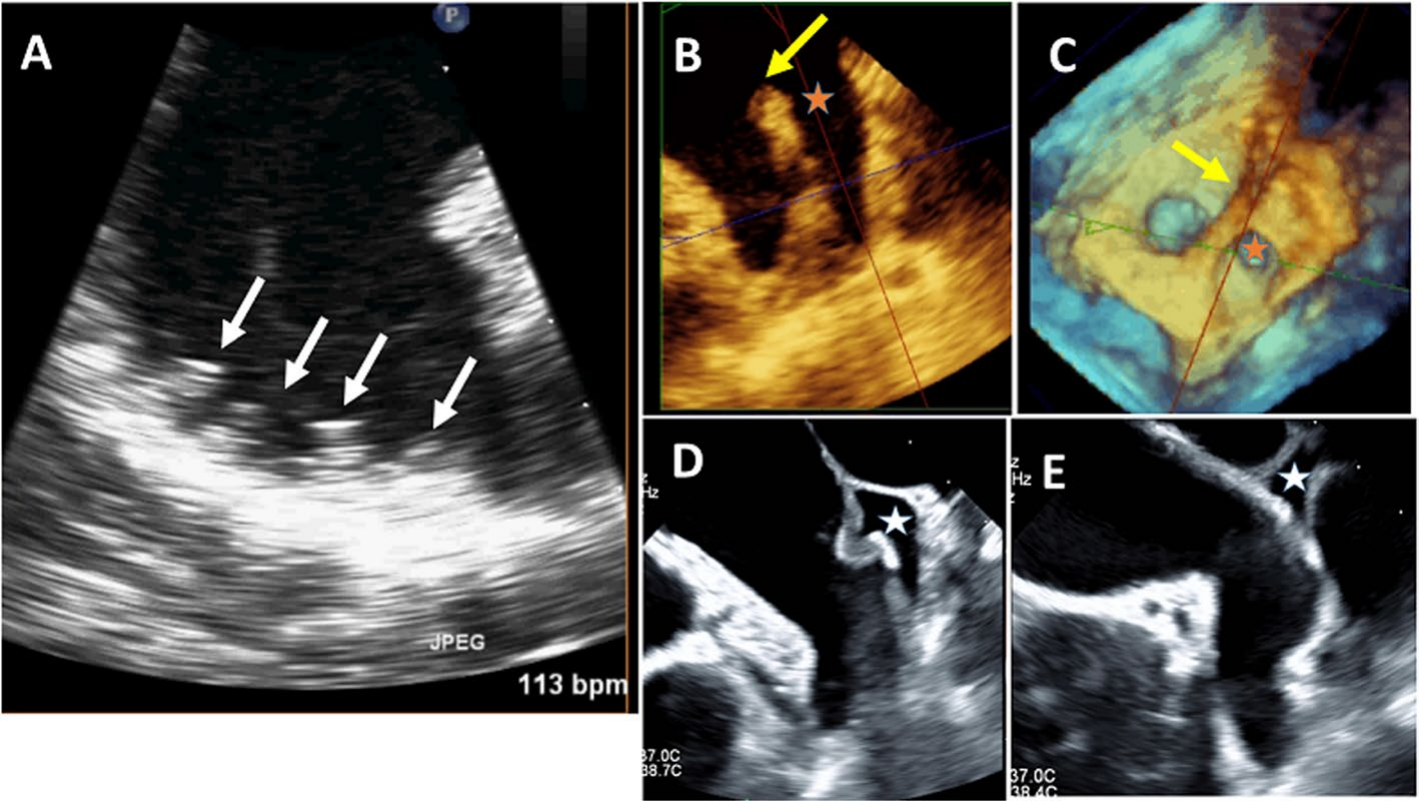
Normal left atrial appendage (LAA) anatomical structures as visualized by two dimensional (2D) transesophageal echocardiography (TEE). AThick pectinate muscle at the apex of the LAA (white arrows), prominent coumadin ridge (yellow arrow) between the LAA and left upper pulmonary vein (red star) as seen by (B) 2D-TEE and (C) 3D-TEE *en face* view. D, E Transverse pericardial sinus (white star) as visualized by 2D-TEE
